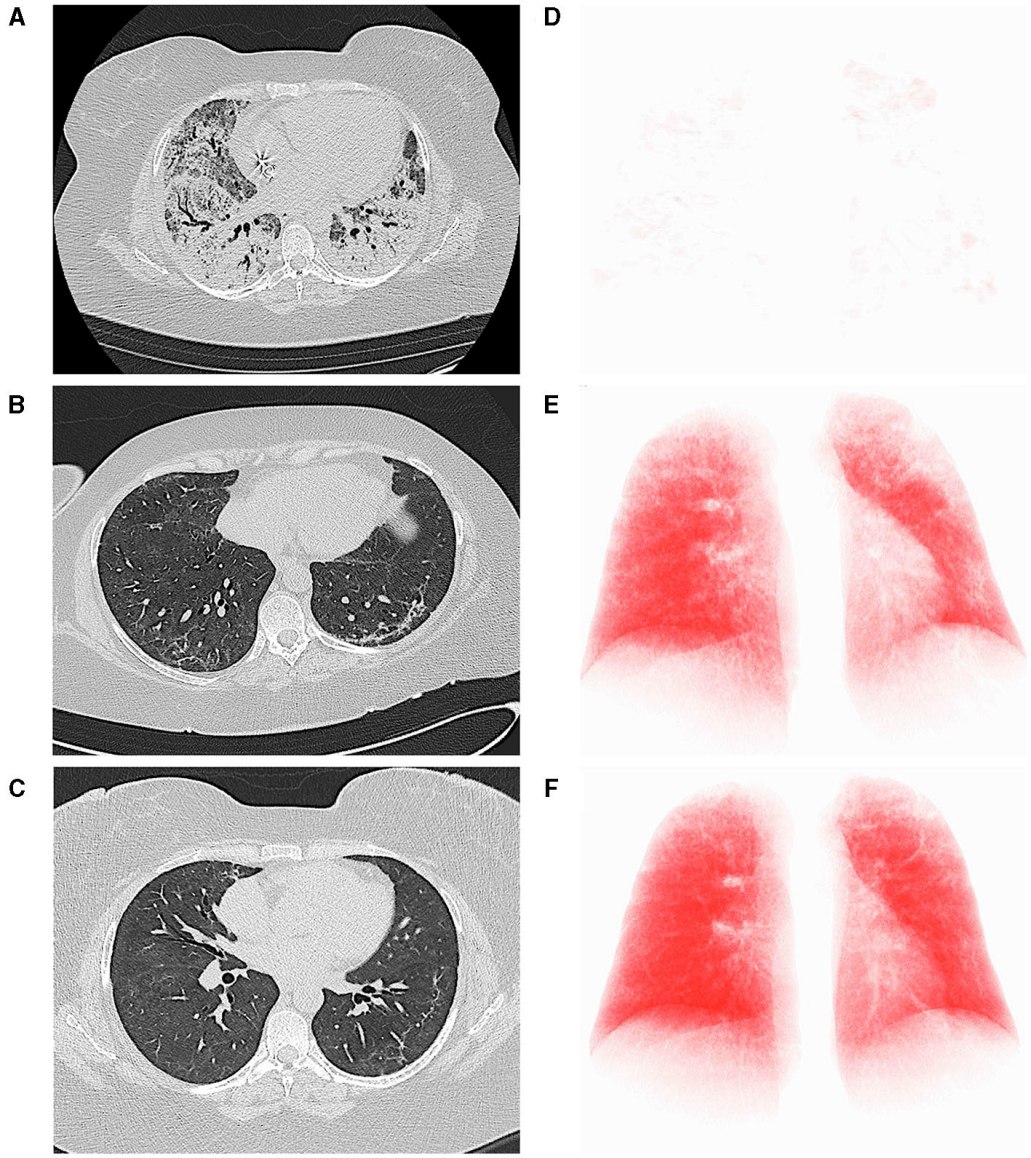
Computed tomography (CT) axial scans (lung parenchyma windowing) acquired, respectively, immediately before (A), 27 (B) and 111 days (C) after the start of nintedanib, demonstrating progressive improvement of lung parenchyma and significant reduction of residual lung damage. At each time point, the axial CT image most representative of the burden of lung involvement was selected. (D–F) report the corresponding three-dimensional volume rendering images (IntelliSpace Portal v.8.0, Philips Medical Systems, Eindhoven, The Netherlands). The red volume represents the well-aerated parenchyma.
